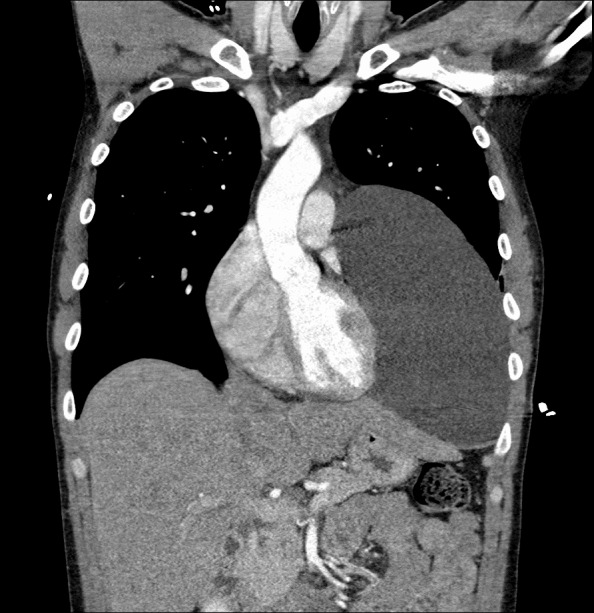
A computed tomography angiogram revealing a large cystic lesion in the left lower and mid-chest abutting the mediastinum and left heart border measuring 20 × 11 × 17 centimeters. The arrows outline the mass.
